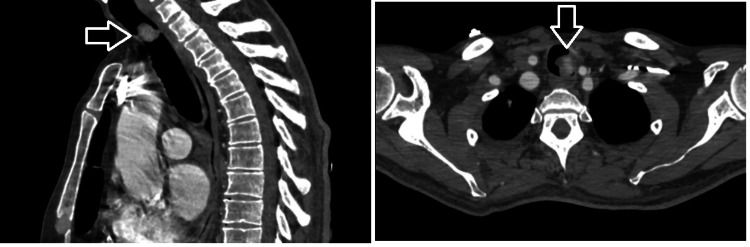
Preoperative thorax computed tomography of patient Sagittal and axial thoracic computed tomography scans demonstrating a mass lesion (arrow) in the upper trachea.
